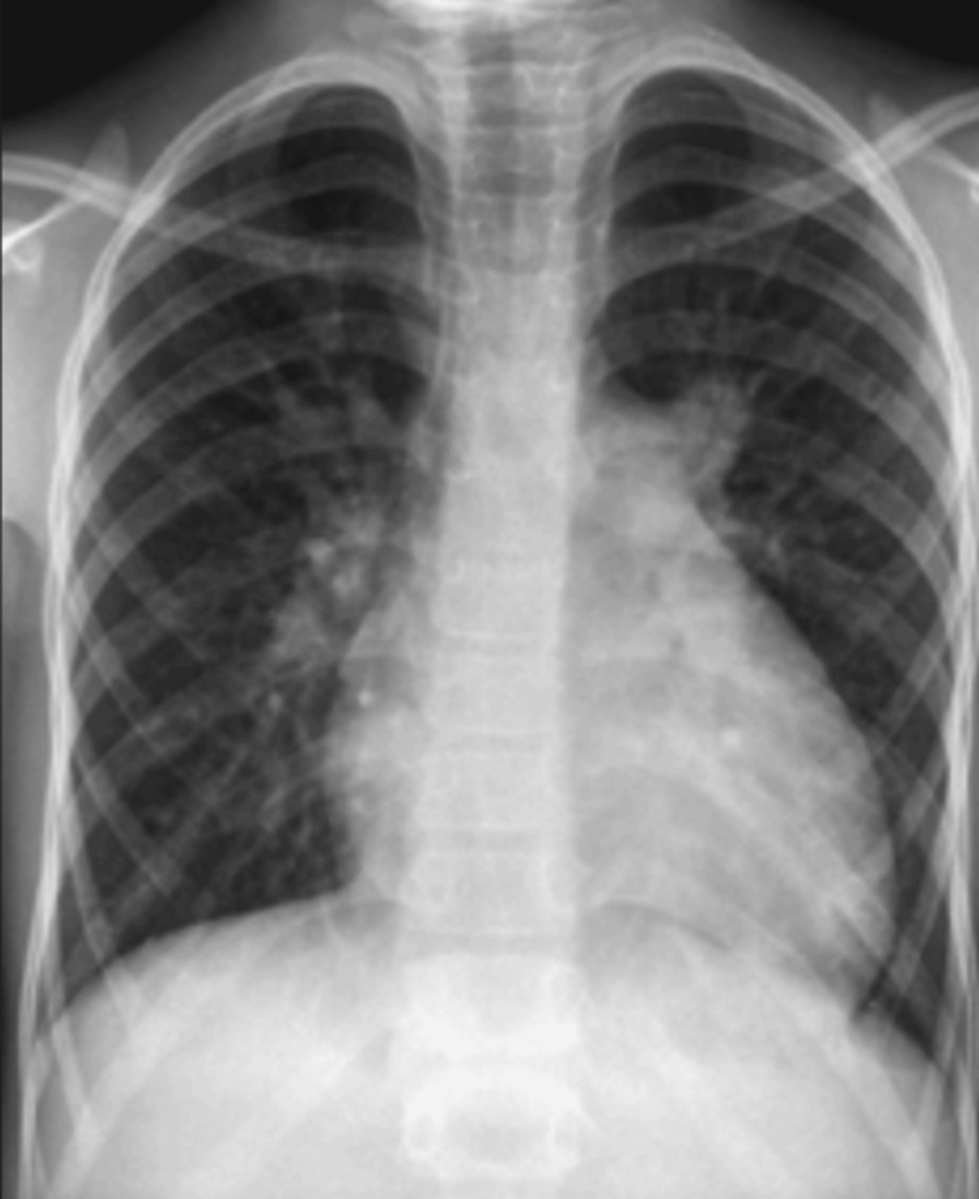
The posterior-anterior chest x-ray of the patient showing dilated right atrium with prominent pulmonary arteries.
